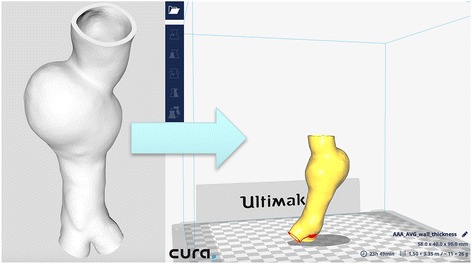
Picture of the 3D reconstruction from the patient’s AAA geometry. The picture was obtained from CT scan data, which was transferred to the software Cura (version 2.5, Ultimaker, Geldermalsen, Netherlands) for layer slicing and setting the 3D printing parameters
